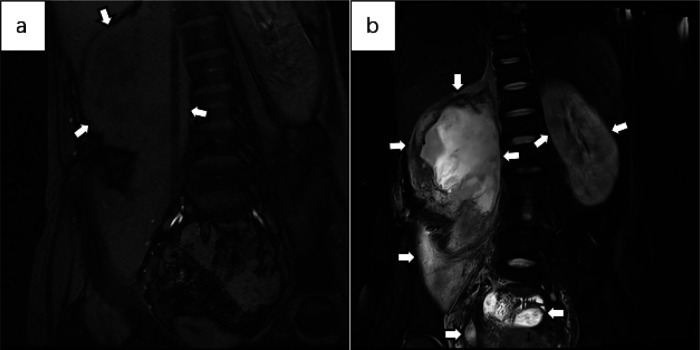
(a) the abdominal MRI T1WI fat-saturated coronal image shows an irregular shape of the right kidney, with the renal cortex noticeably thickened and uneven. The renal calyx and pelvis appear with slightly lower signal intensity. The lesion extends to the perirenal area and the psoas major muscle, suggesting the formation of a psoas major abscess. There is no obvious abnormality in the shape or size of the left kidney. (b) The MRI T2WI fat-suppressed image in the coronal view shows patchy high signal areas in the perirenal region and renal pelvis on the right side, suggesting TB pyonephrosis with the formation of a psoas abscess. The left kidney is slightly enlarged, with possible edema. Low signal areas are seen in the renal parenchyma, consistent with early signs of renal TB. Abnormal high signal areas are observed in the pelvis, which, combined with the patient's medical history, suggest pelvic TB.
